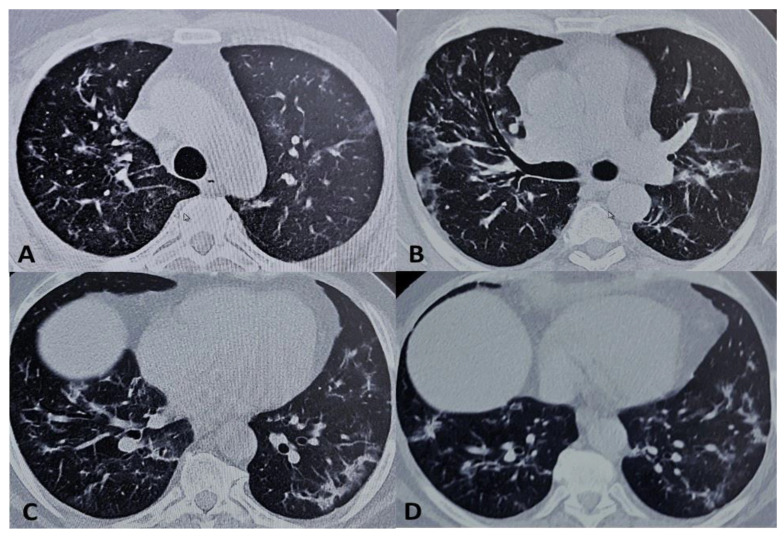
Computerized tomography (CT) scans after 96 h. Compared to pretreatment CT scans (Figure 1), (A–D) thin-slice (1 mm) axial unenhanced CT images showed an evident reduction (in terms of extension and density) of the bilateral lung interstitial infiltrates as well as of the extensive multiple patchy ground-glass opacities. Lymphadenopathy was also reduced.
